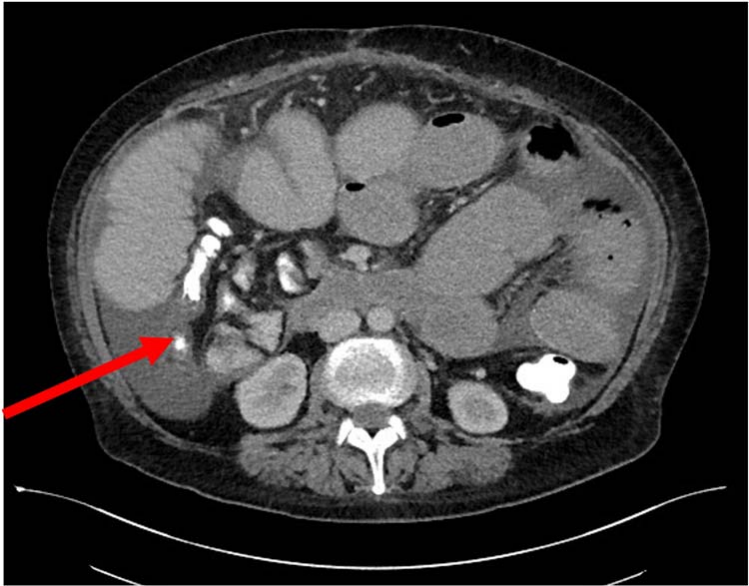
Axial CT of SBO secondary to internal hernia.
